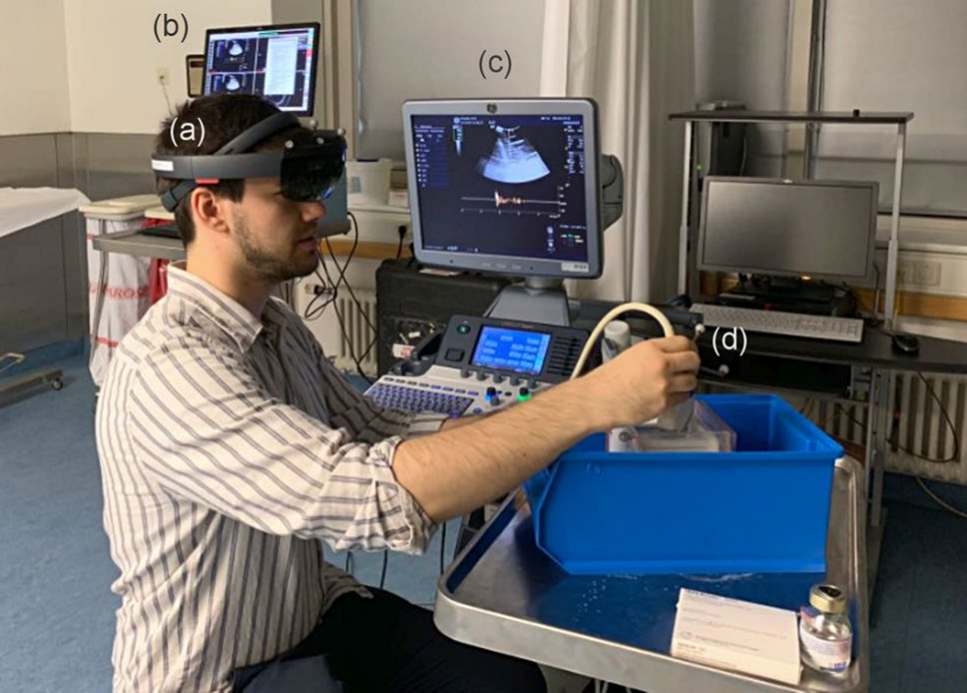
Exemplary use of a AR-HMD, b surgical navigation system, c ultrasound system, and d transducer
